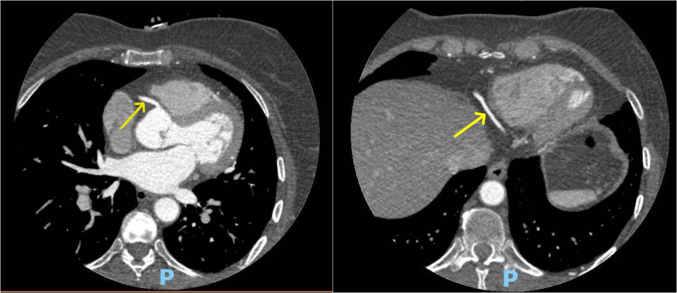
Coronary Computed Tomography Angiography Showing No Soft or Calcified Plaques in the RCA (yellow arrow) RCA = right coronary artery.
